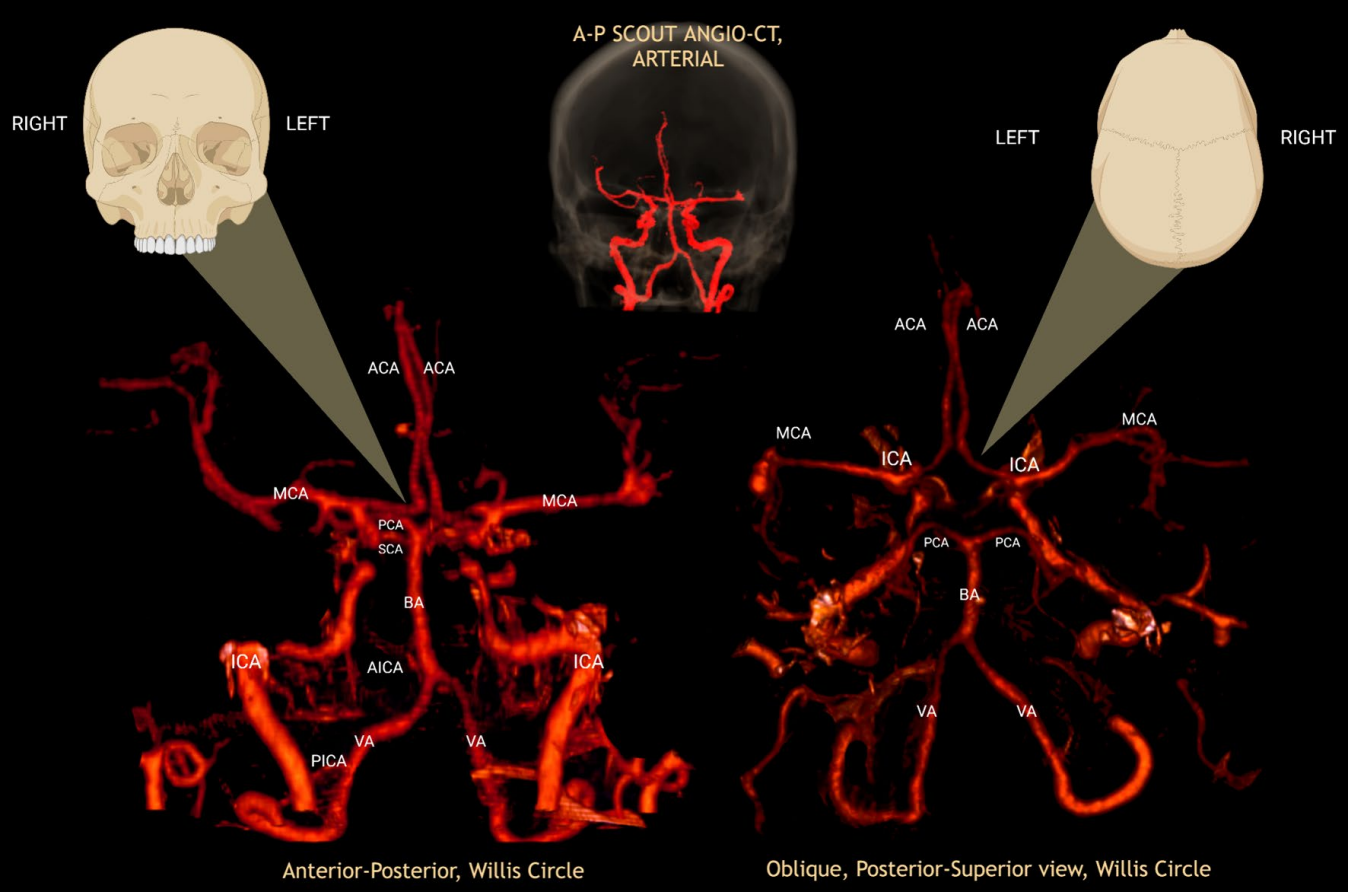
Angio-CT 3D render of arterial circulation of the brain, comprised by anterior and posterior circuits, with detailed view and labeling of the main vessels. In the oblique posterior-superior view, it is observed incidentally a dominant right Posterior Communicating Artery with a much greater caliber than its counterpart. *ACA* Anterior cerebral artery, *AICA* Anterior inferior cerebellar artery, *MCA* Middle cerebral artery, *PCA* Posterior cerebral artery, *PICA* Posterior inferior cerebellar artery, *SCA* Superior cerebellar artery, *VA* Vertebral Artery
